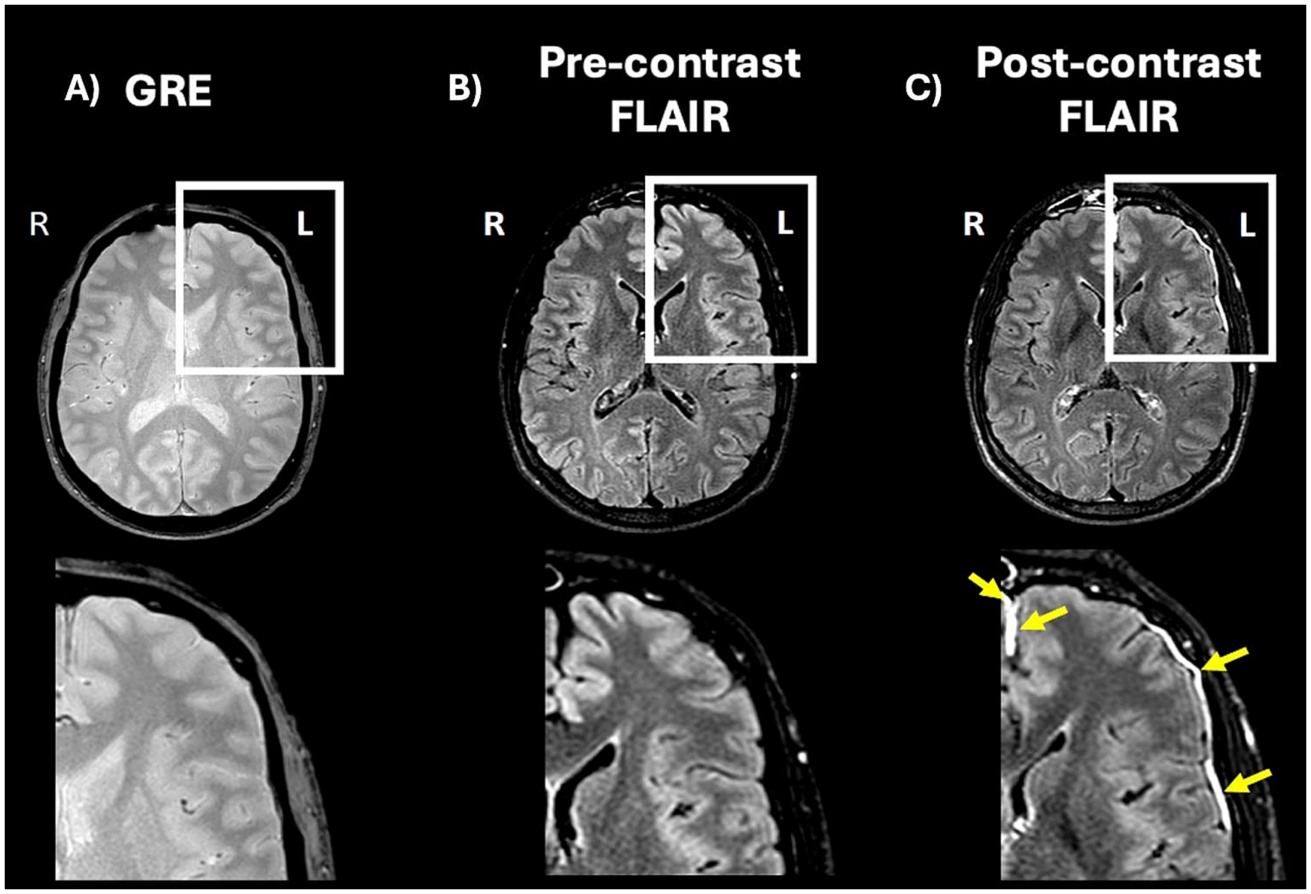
Axial MRI scans from a patient with traumatic brain injury demonstrating the absence of subdural hematoma (SDH) but presence of traumatic meningeal enhancement (TME). (A) Gradient echo (GRE) sequence shows no evidence of hemorrhage. (B) Pre-contrast fluid attenuated inversion recovery (FLAIR) sequence shows no abnormal meningeal signal. (C) Post-contrast FLAIR sequence reveals hyperintense enhancement along the left convexity (yellow arrows), consistent with TME in the absence of visible SDH. These findings highlight that TME can occur independently of hemorrhage and may represent isolated meningeal injury or subtle blood–brain barrier disruption. Adapted from Arbona-Lampaya et al. (15).
